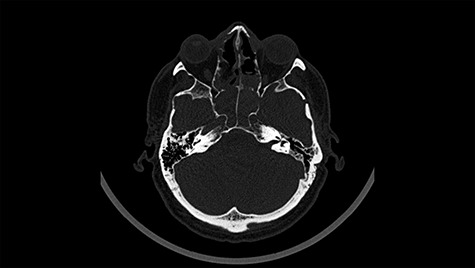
Head CT after polypectomy—seen sphenoid sinus and both maxillary sinuses filled with blood. (Department of Neurosurgery own material).
